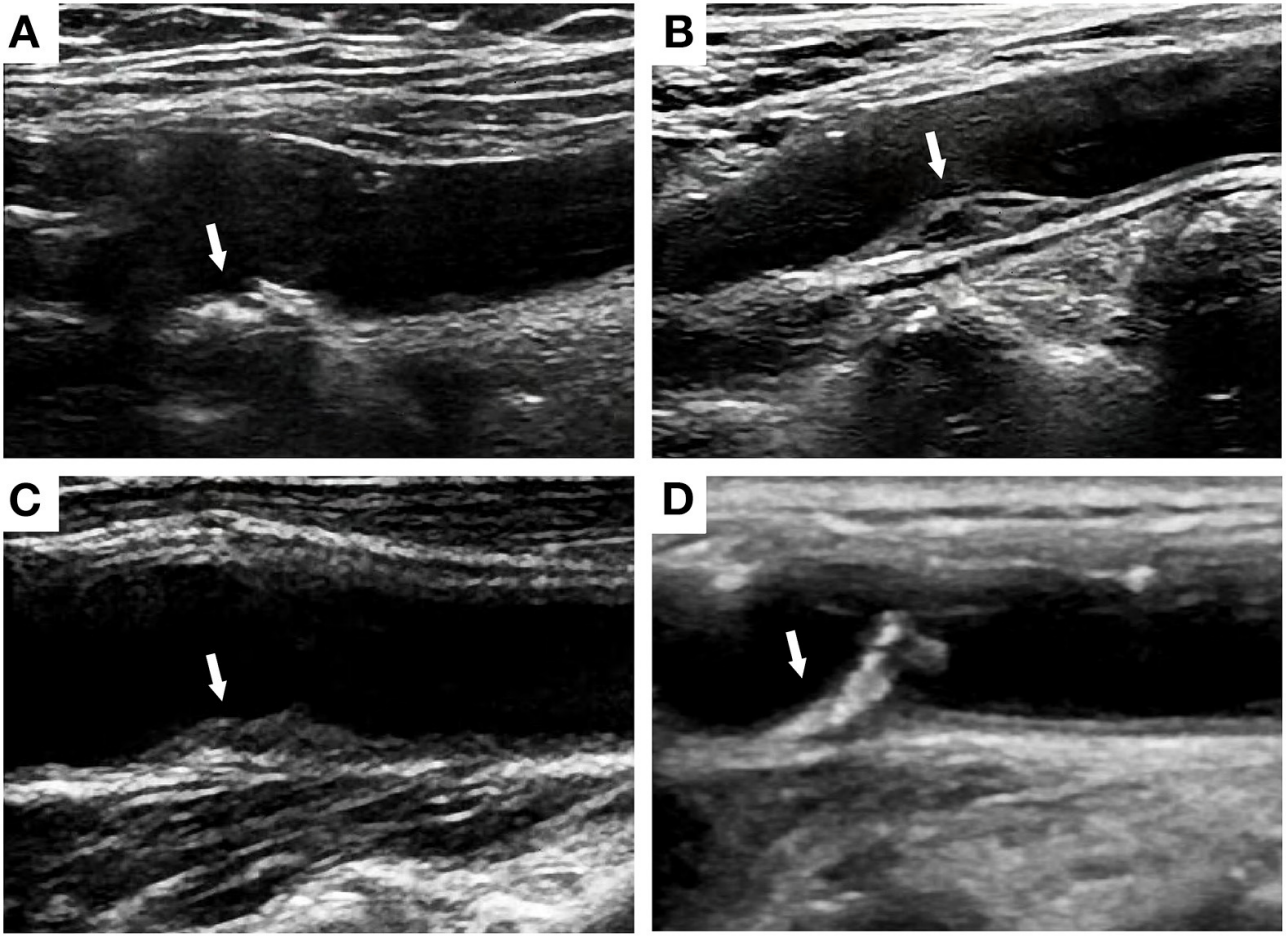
Ultrasound images of hyper-echo (A), low-echo (B), mixed-echo (C), and ulcer plaques (D) at the carotid bulbs.
